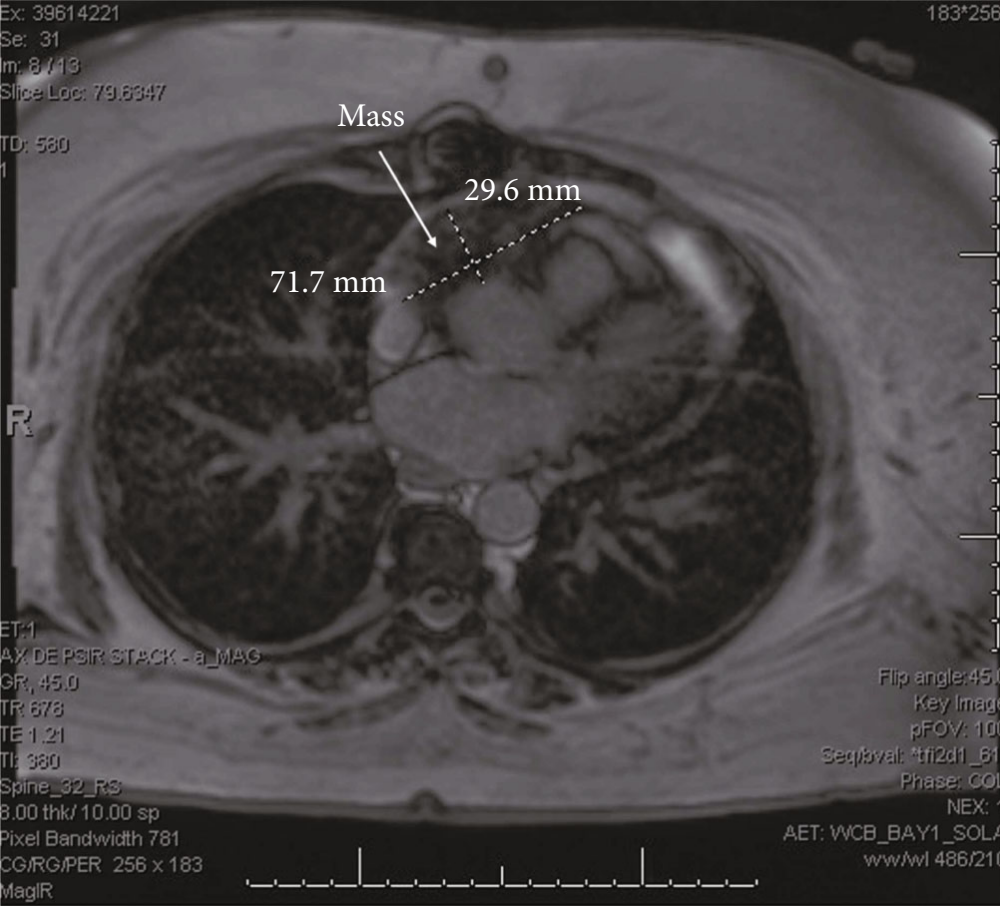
Follow-up magnetic resonance imaging demonstrated decreased size of the lesion posttreatment measuring 2.96 × 7.17 cm.
